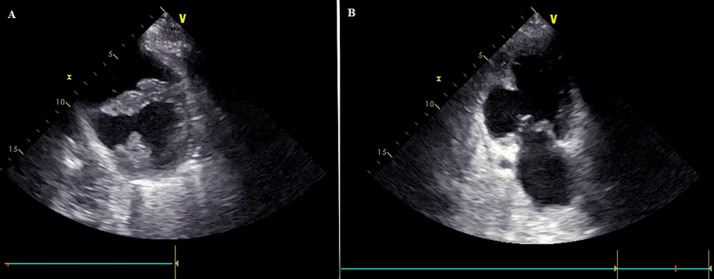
echocardiography findings: A) parasternal short-axis view showing the aneurysm; B) apical 2-chamber view showing the aneurysm
